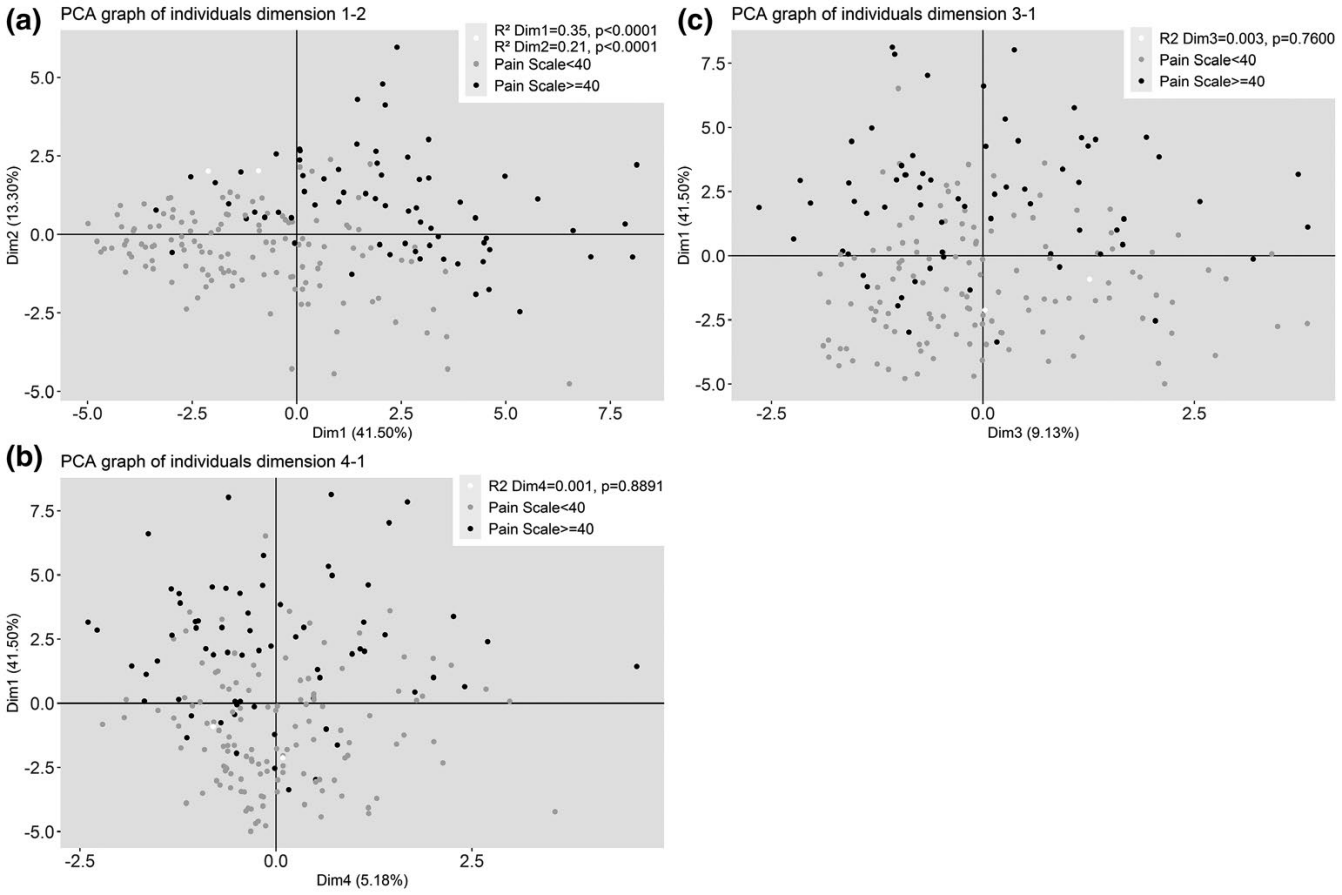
Principal component analysis – Analysis of Variance on the different patient coordinates on PCA axes in relation to their pain level (VAS < 40 mm/100 mm; VAS ≥ 40 mm/100 mm); dim 1: dimension 1 or axis 1 (emotional impact: anxiety and depression scores, sensory and emotional qualifier scores, HAQ, impact of the pain in daily life); dim 2: dimension 2 or axis 2 (Disease activity score DAS28, pain relief with current treatments); dim 3: dimension 3 (duration of RA, SENS radiographic score); dim 4: dimension 4 (ESR, DAS28) (*N* = 197)
